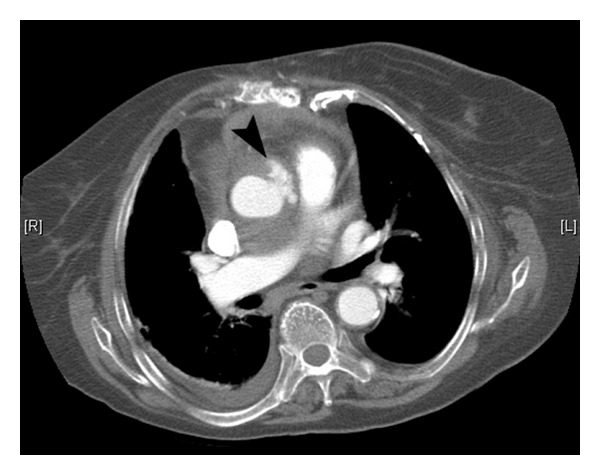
Axial slice from contrast-enhanced CT study demonstrating direct communication and erosion of the abscess cavity into the anterior ascending aorta resulting in a localised peri-aortic area of contrast extravasation (black arrowhead).
